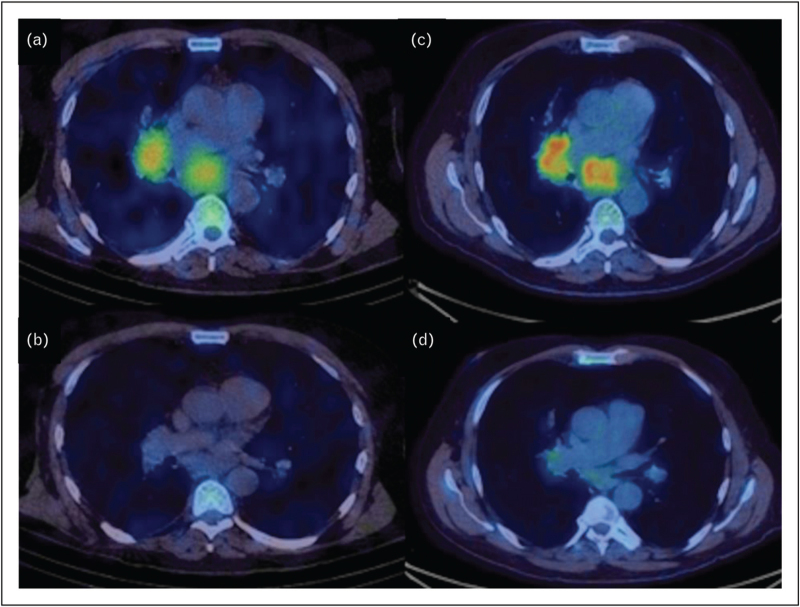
[^99m^Tc]NM01 (a PD-L1 targeting nanobody) showing high PD-L1 expression in non-small cell lung cancer right hilar and subcarinal nodes before treatment with pembrolizumab (a), and [^99m^Tc]NM01 scan at 9 weeks showing reduction in PD-L1-expressing tumour (b). This is compared with [^18^F]FDG PET demonstrating high tumour uptake (c) with a significant 9-week metabolic response (d) as predicted by high PD-L1 baseline expression.
